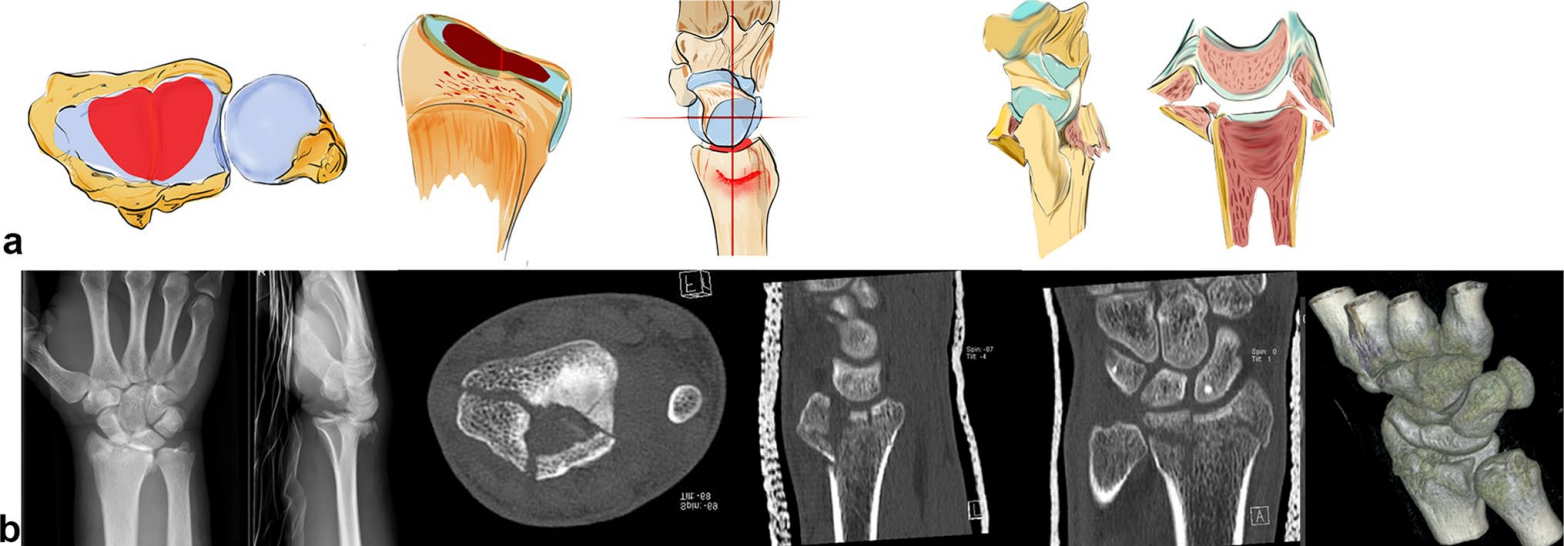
A central impression can occur with or without a dorsal or palmar fracture of the cortical bone. In these cases, the advantages of the CT scan are evident (a + b)
